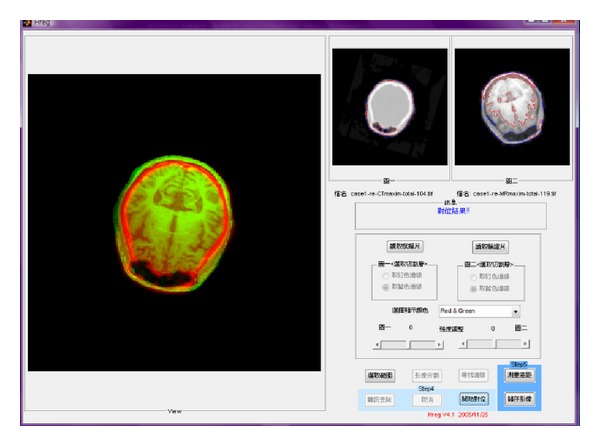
An example of registration of the CT image (middle small panel) and the MR image (right small panel) to render the final fused image (left large panel). The red and blue lines in the small panels are the detected boundaries and the contour of the head.
